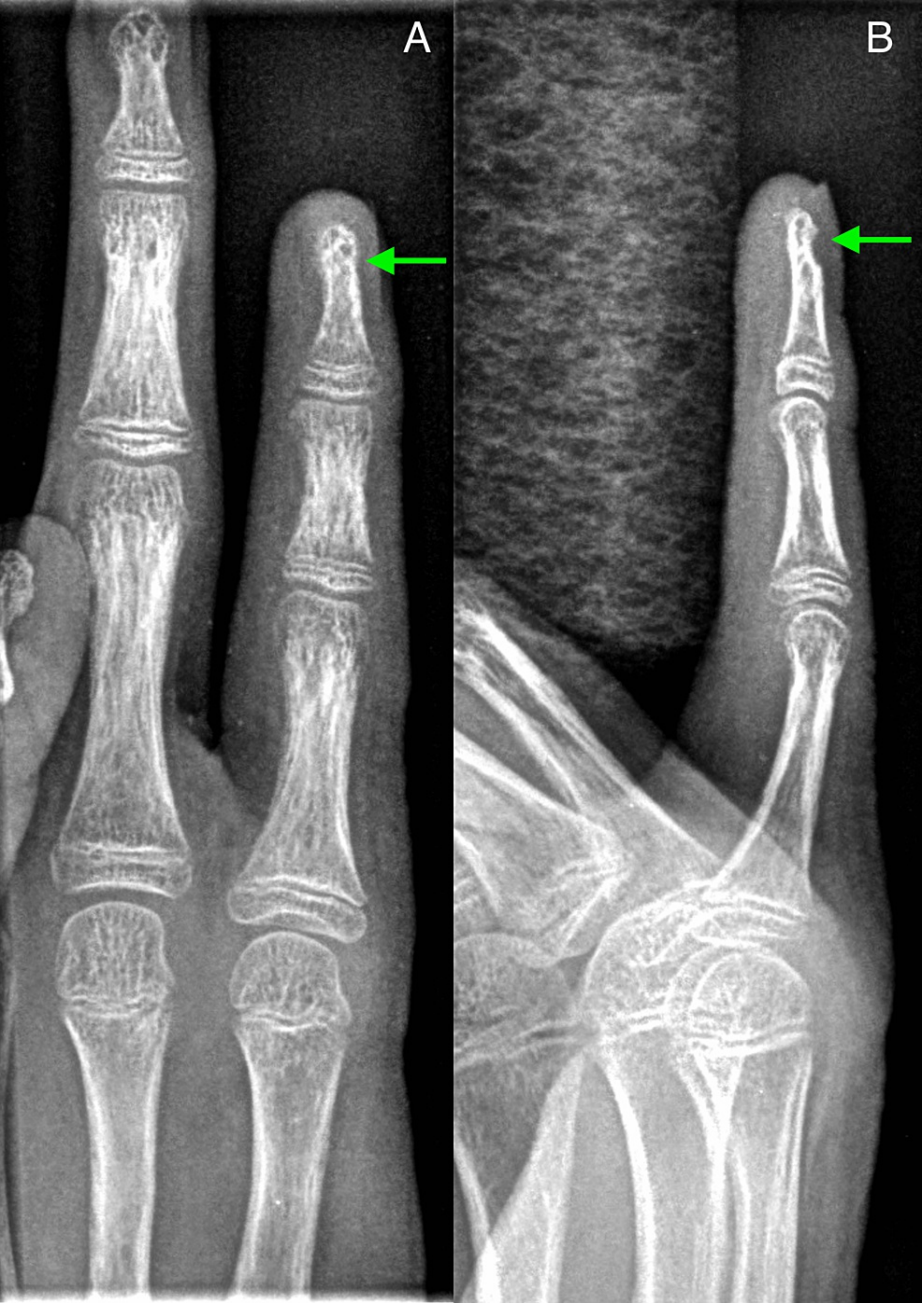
Initial radiographic evaluation of the little finger. Initial anteroposterior (A) and lateral (B) radiographs of the little finger demonstrate cortical scalloping underlying the nailbed (green arrow). Note how this is best appreciated on the lateral view and not visualized on the anteroposterior view. There is no appreciable soft tissue edema or radio-opaque foreign body.
